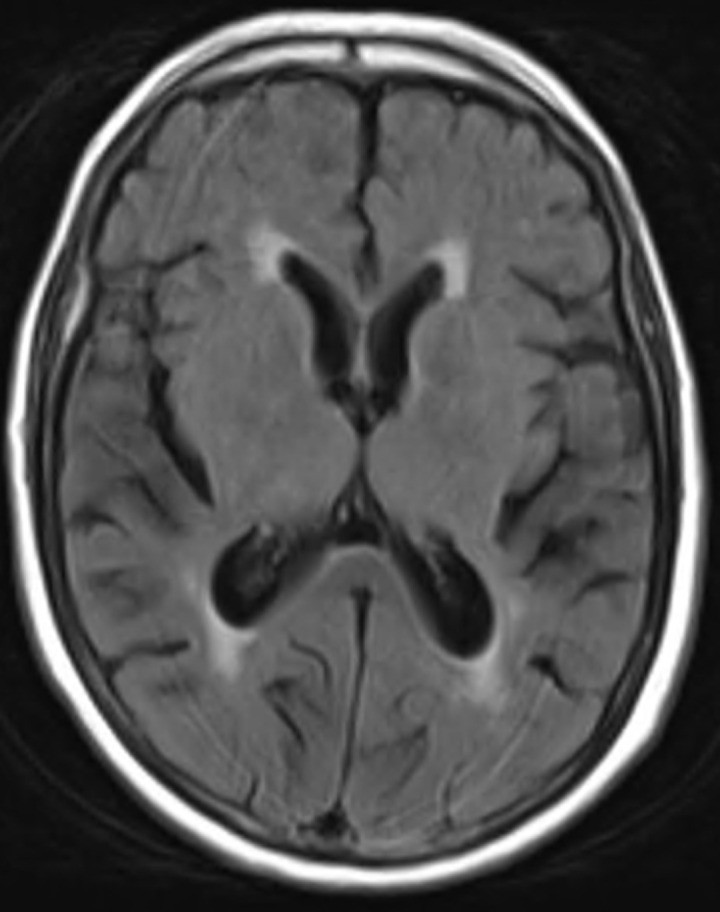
normal axial brain MRI serie 7 T2 Flair
